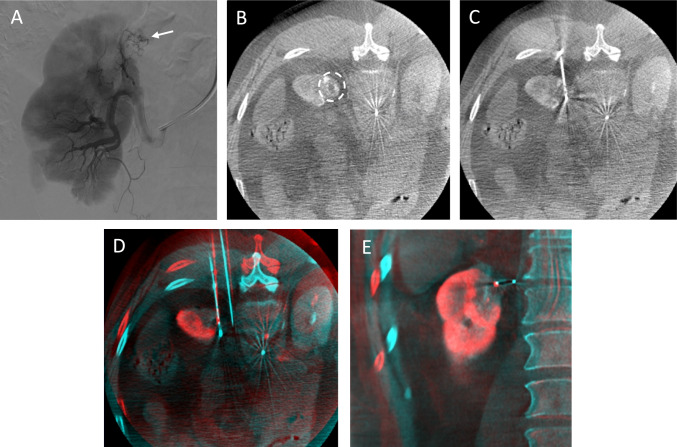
Example of RenACAGA ablation of a tumor in the upper pole of the left kidney. A Digital subtraction angiography of the left renal artery shows the target lesion (white arrow); B The target lesion (white circle) visualized with C-arm CT renal arteriography (C-arm CTRA); C Ablation needle in position; D and E Pre- and post-ablation C-arm CTRAs are fused with XperGuide software (Philips) in axial and coronal planes, showing adequate ablation margins
